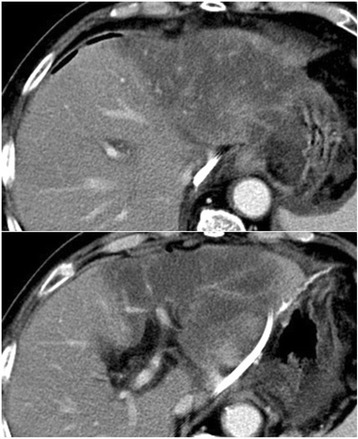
Case 1 CT findings 5 days after pancreaticoduodenectomy. Extensive necrosis is observed in the lateral segment of the liver
